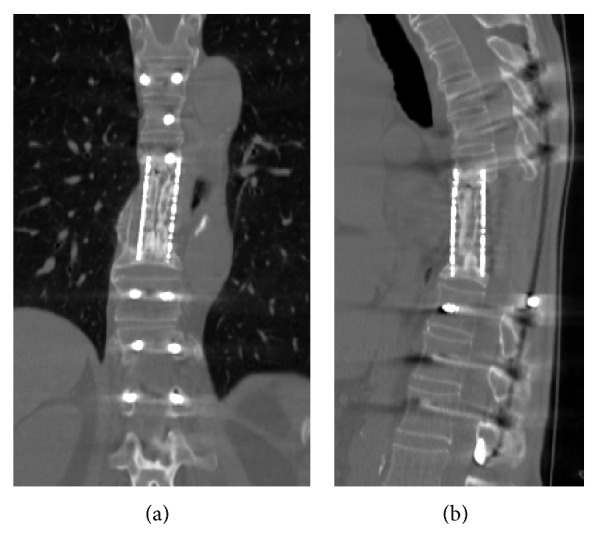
CT scans at 20 months after operation showing bone union. (a) Coronal, (b) sagittal.
